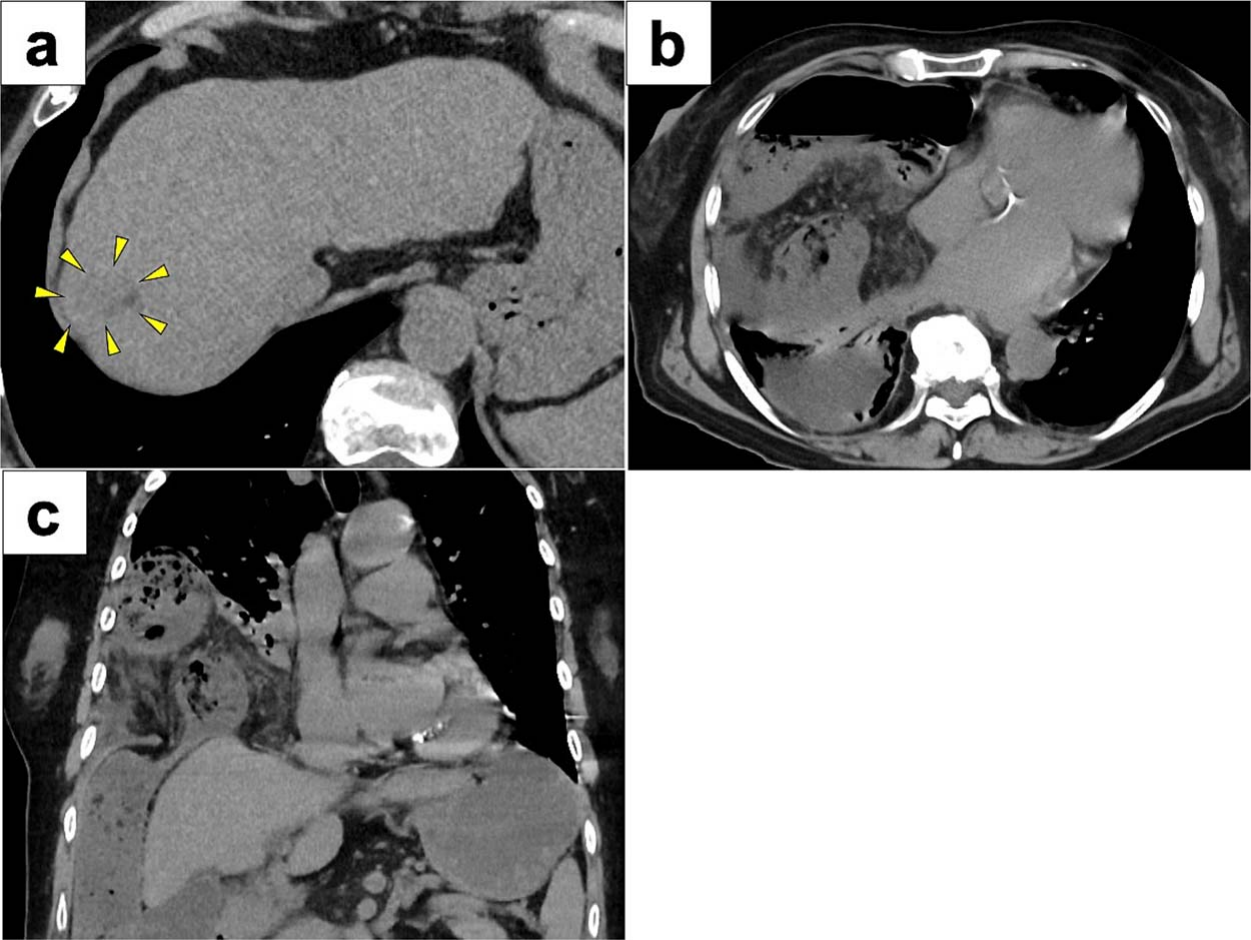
CT images of preoperative examinations. (a) Pre-RFA CT showing that HCC was a 20-mm lesion located in the subcapsular portion of hepatic Segment 8 (arrow). (b and c) Axial and coronal abdominal CT showing incarceration of the transverse colon into the thoracic cavity.
